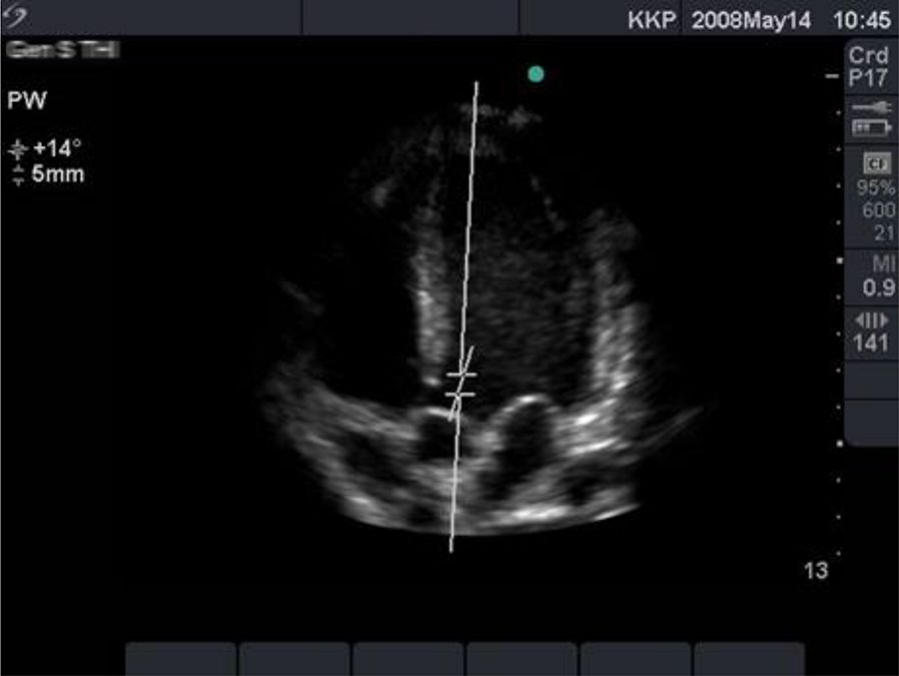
Apical 5 chamber view with pulsed wave Doppler sample volume
